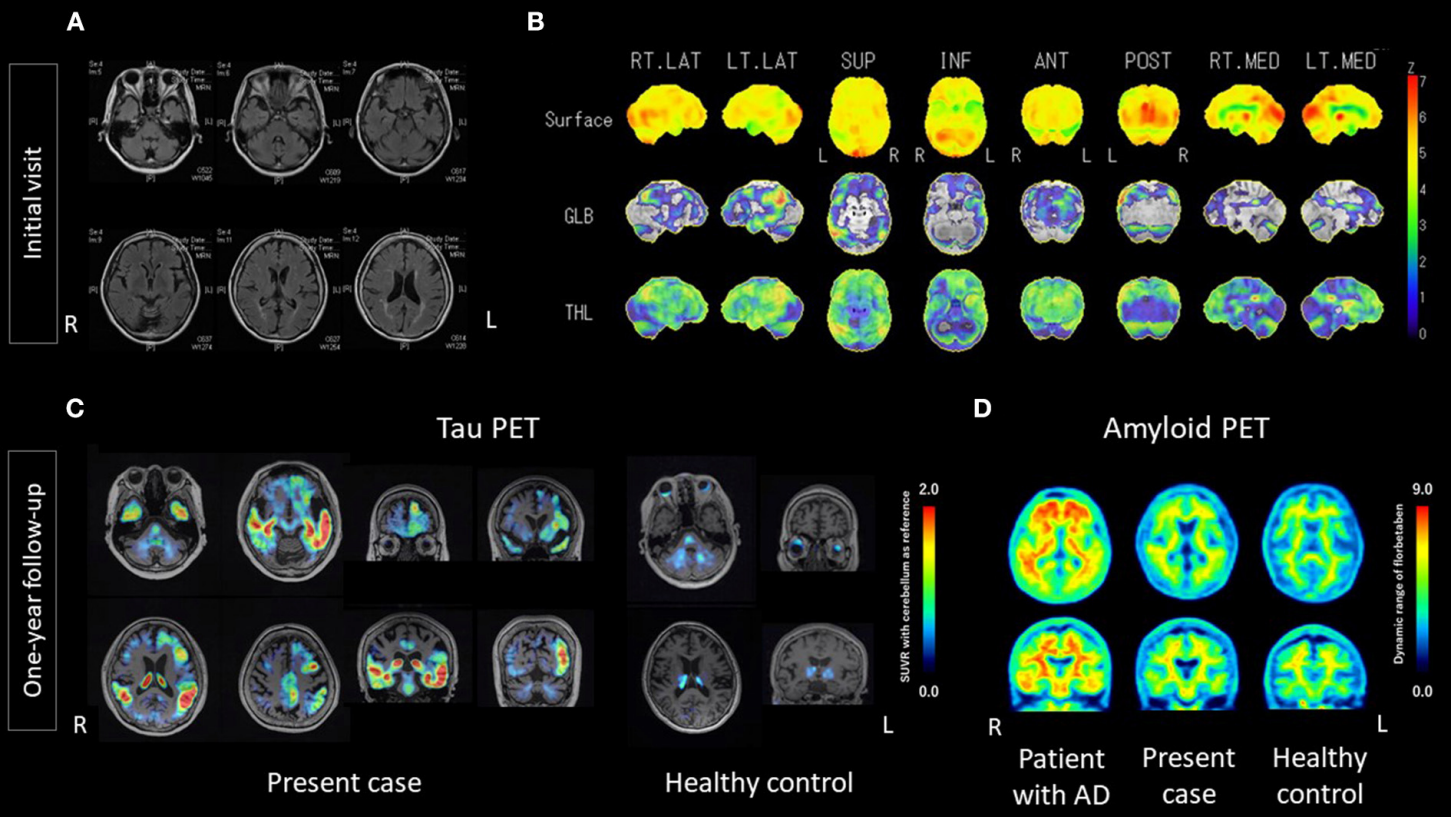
Results of MRI, 3D-SSP of SPECT, ^18^F-florzolotau tau PET, and ^18^F-florbetaben amyloid PET. (A) MRI at the initial visit showed mild atrophy of the cerebrum, with a left predominance, and of the cerebellum. Hyperintense signals in the white matter suggest old lacunar infarctions and/or chronic ischemic changes. (B) 3D-SSP of SPECT at the initial visit showed a mild decrease in blood flow in the posterior cingulate gyrus, precuneus and cerebellum. (C)
